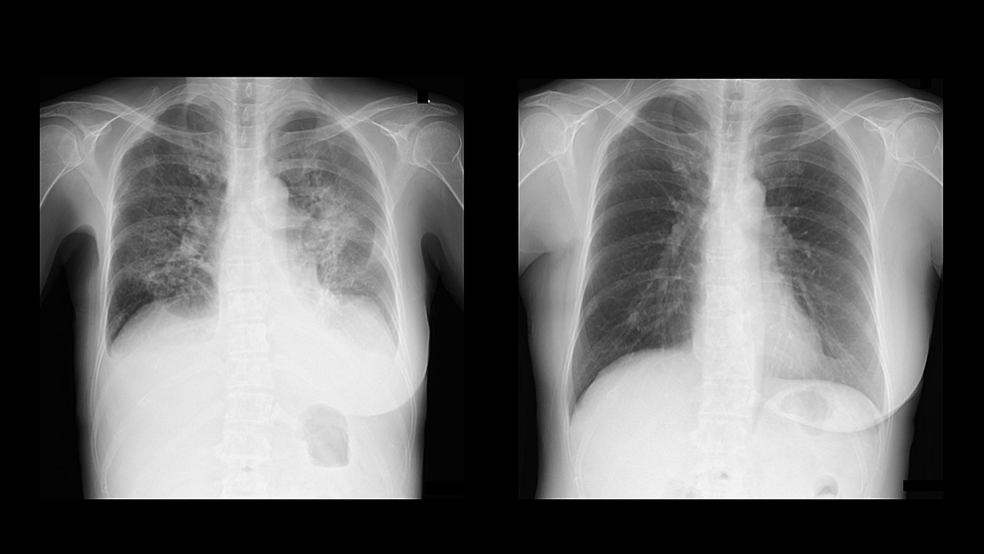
Chest X-ray before and after treatment. Left: chest X-ray on admission revealed remarkable pulmonary congestion. Right: chest X-ray after eight months.
